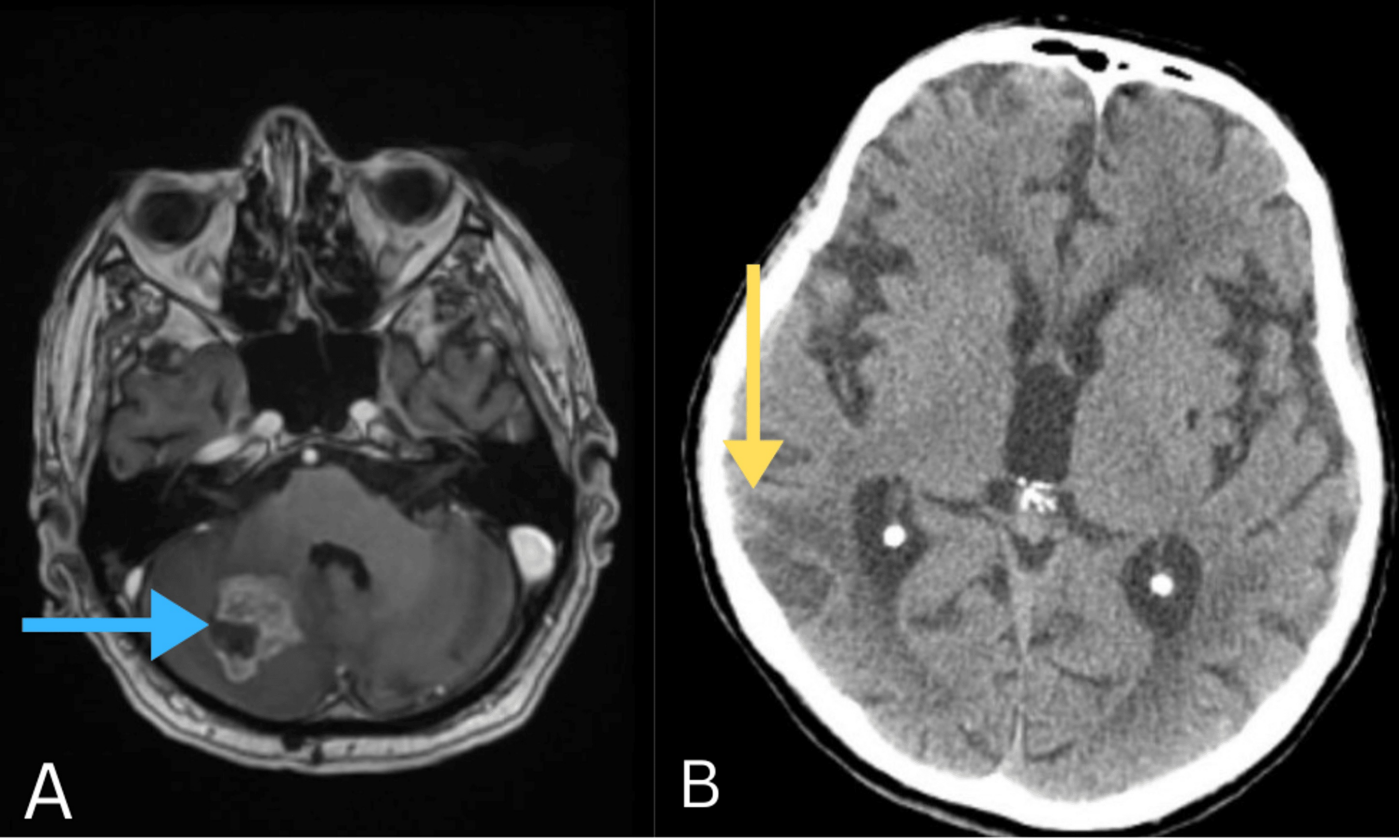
MRI an CT scan. (A) MRI axial 3DT1: the presence of brain metastasis in the right cerebellar hemisphere (blue arrow); (B) CT axial: at the level of the right parietal lobe, a dense heterogeneous mass with a perilesional mass effect and peripheral edema in the vicinity (yellow arrow).
